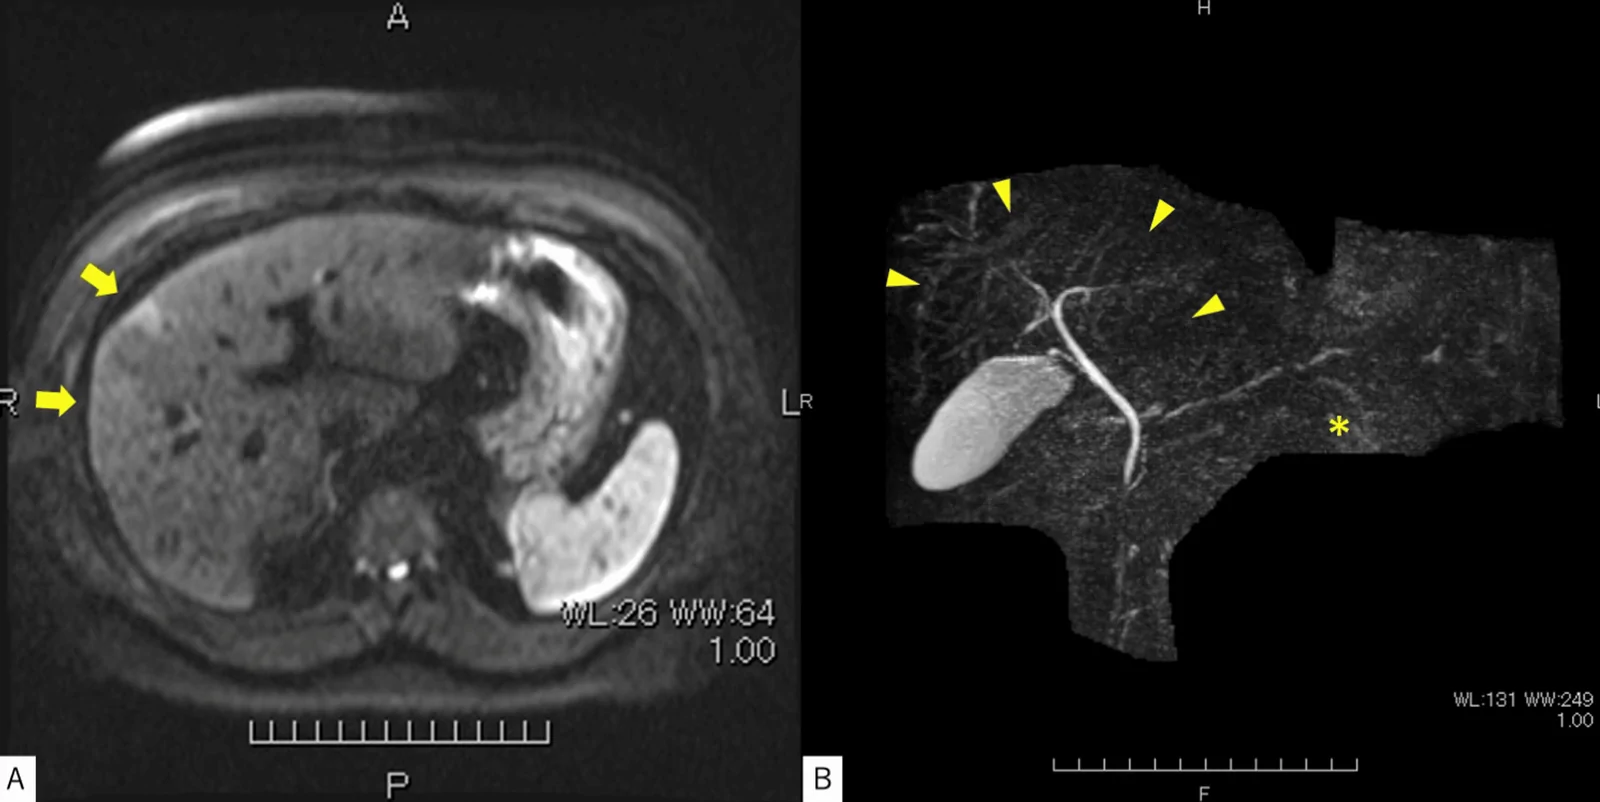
Follow-up magnetic resonance imaging and MRCP findings after steroid therapy (at Week 30 of steroid therapy) (A) Axial diffusion-weighted imaging showing minor residual hyperintensity in the hepatic parenchyma (arrows). (B) Follow-up MRCP demonstrating marked improvement of intrahepatic bile duct dilations and strictures (arrowheads), as well as the complete disappearance of the previously noted cystic lesion in the pancreatic tail (asterisk) MRCP: magnetic resonance cholangiopancreatography
